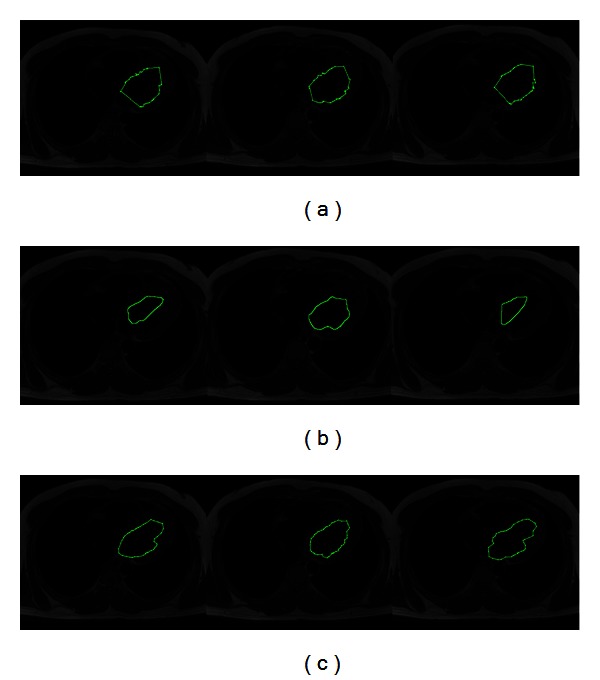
MR images (human left ventricle segmentation): (a) results of traditional ACM, (b) results of graph cut method, and (c) results of MACPSO implementation.
